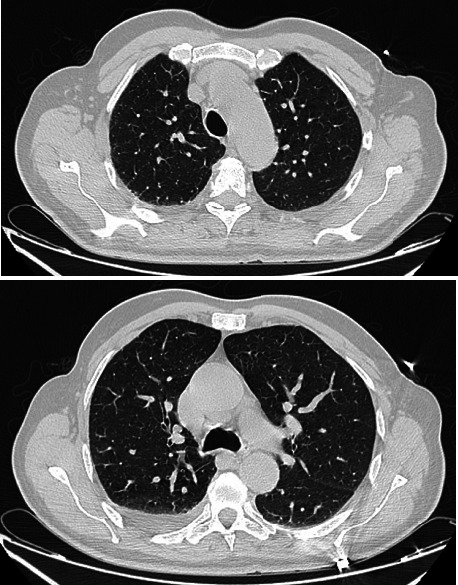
Axial CT-scan views showing bronchial wall thickening associated to a small right pleural effusion.
